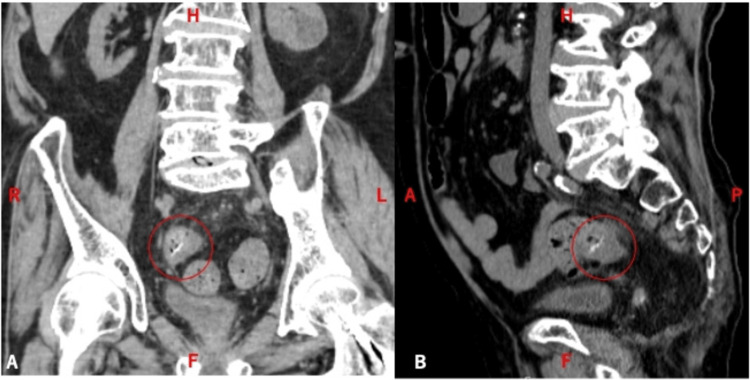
(A) Coronal and (B) sagittal CT showing a linear high-attenuation structure in the rectosigmoid colon (circle) CT: computed tomography
